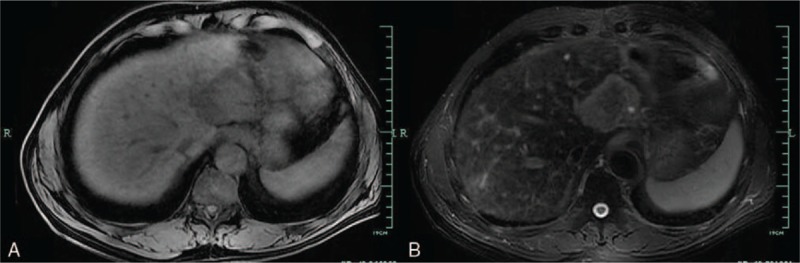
Magnetic resonance imaging shows a lesion with slightly low-signal intensity on T1-weighted imaging (A) and slightly high signal intensity on T2-weighted imaging (B).
